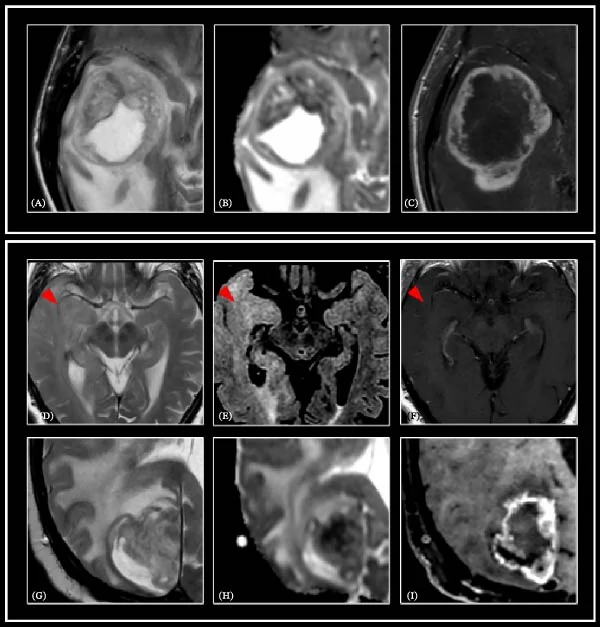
Case 1: oval‐shaped lesion in the right fronto‐temporal region, surrounded by extensive perilesional edema. The lesion shows a central necrotic component and a peripheral solid component. After Gadolinium administration, an irregular and peripheral enhancement of the lesion is observed. (A) Axial fast spin echo T2‐weighted images; (B) apparent diffusion coefficient (ADC) maps; (C) axial spin echo post‐contrast T1‐weighted images. Case 2: Baseline MRI (D, E) infiltrative lesion (red arrowhead), hyperintense on T2‐weighted images, involving both the cortex and white matter in the right temporo‐occipital and parietal region, without restriction on the ADC map. No pathological contrast enhancement is observed after Gadolinium administration (F). Recurrent case at the follow‐up MRI: note the global increase in the extent of the infiltrative lesion, with signs of tissue swelling. Evidence of a newly appearing solid component in the right temporo‐occipital region, with heterogeneous signal on T2‐weighted sequences (G), apparent diffusion coefficient (ADC) maps (H); after gadolinium administration, an intense enhancement of the solid part of the tumor is detected (I).
